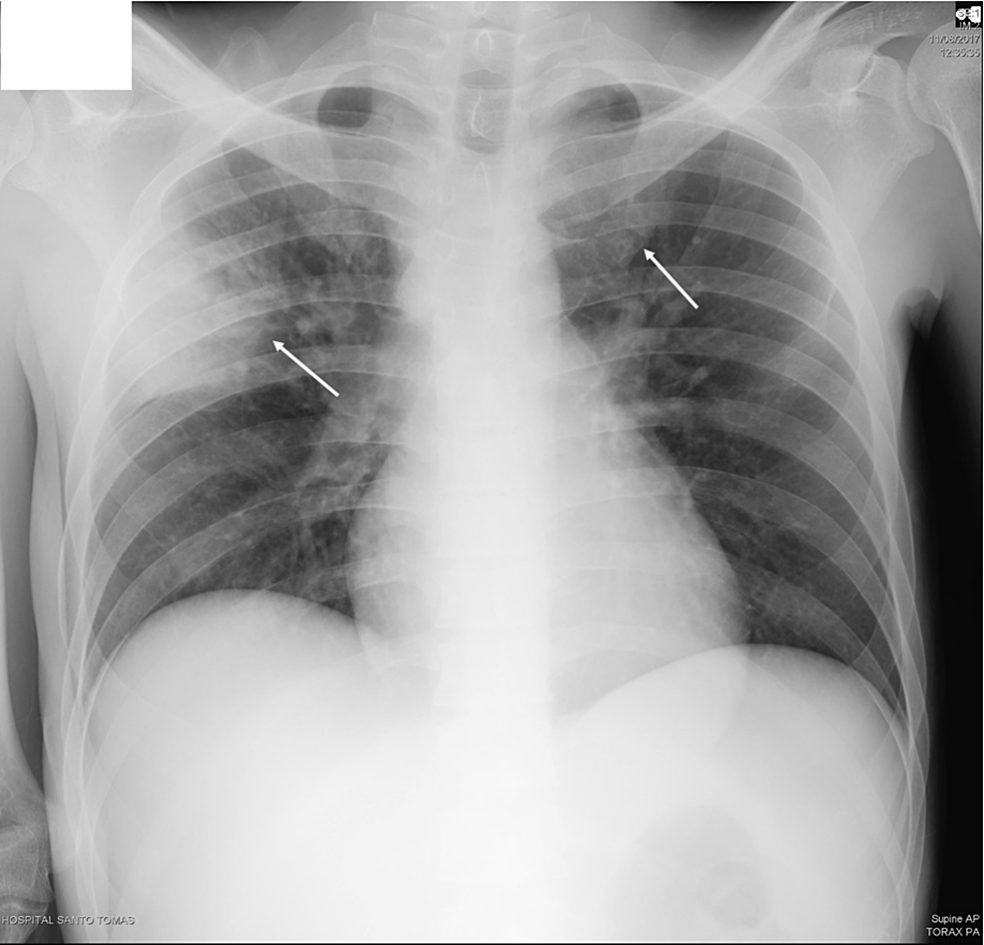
Chest x-ray on day 46 of hospitalization Image showing the chest x-ray after positive liver biopsy for *Mycobacterium tuberculosis*. The arrows show left lung alveolar infiltrates in the midpart of the lung and apical alveolar infiltrates in the right lobe.
